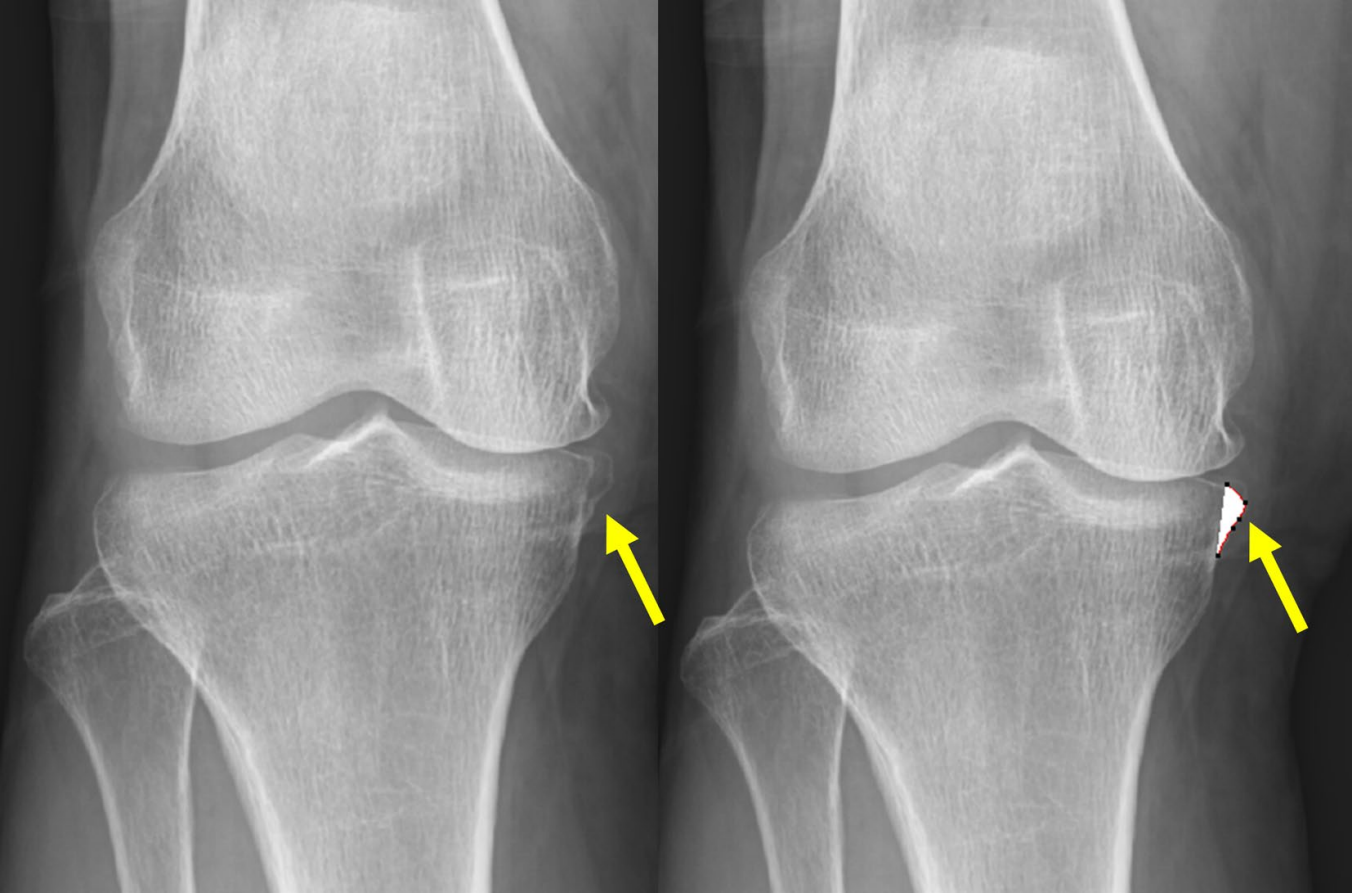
Representative knee frontal radiograph showing the measurement of osteophyte area using the KOACAD system. The system detects the bone margin and calculates the osteophyte area (outlined by the red line) as the medially prominent bony outgrowth beyond the extended contour of the tibia. Arrowhead indicates the osteophyte.
